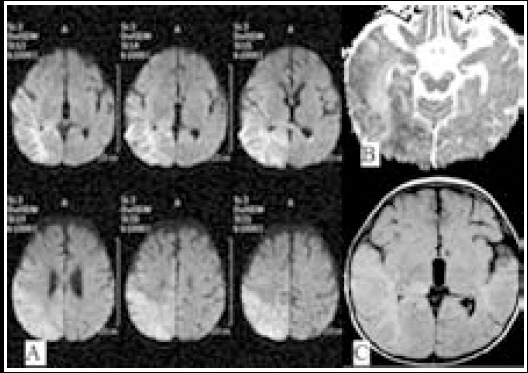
3 year-old male patient, on the 5th day of admission: On DWI (image A), ADC series (image B) and FLAIR image (image C) restricted diffusion on the right hemisphere involving cortico-subcortical and deep white mater areas. Hyperintense signal is observed in FLAIR serial but conspicuity of signal alterations is best depicted on DWI due to the early examination (secondary to predominance of cytotoxic edema).
